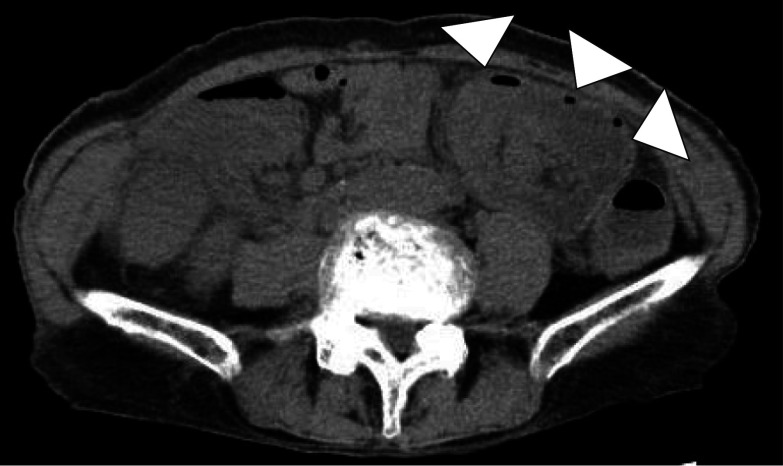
CT image of thorax and abdomen. The lesion was located in the transverse colon and was intussuscepted to the small intestine (arrows)
